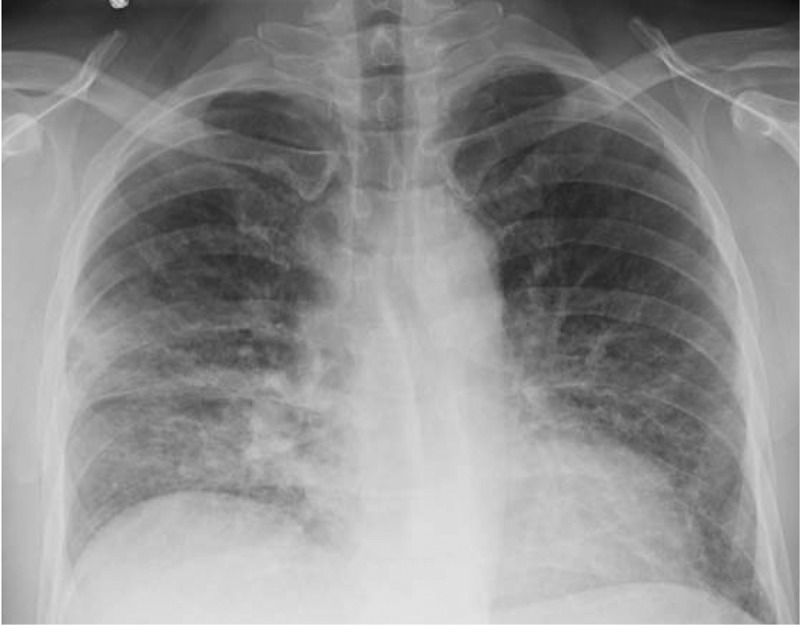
Chest radiograph done on day 6 shows improvement in bilateral infiltrates.
